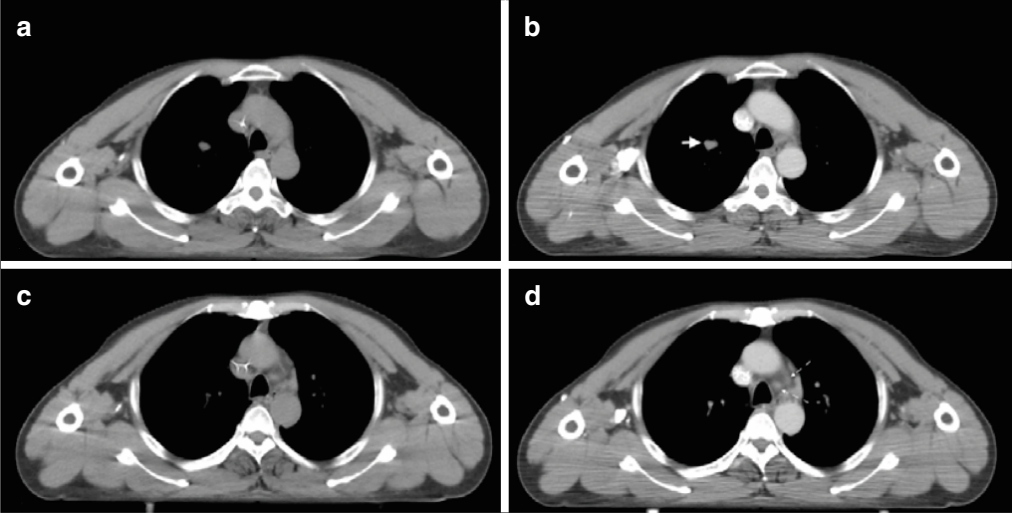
The Hounsfield units (HUs) of lesion in plain 3D-CT (a) and CE-4D-CT (b) were 13 ± 3 and 42 ± 7, respectively; and the HUs of mediastinal lymph nodes in plain 3DCT (c) and CE-4D-CT (d) were 1 ± 25 and 17 ± 26, respectively. The nodes were clearly identified (*dotted arrow*)
